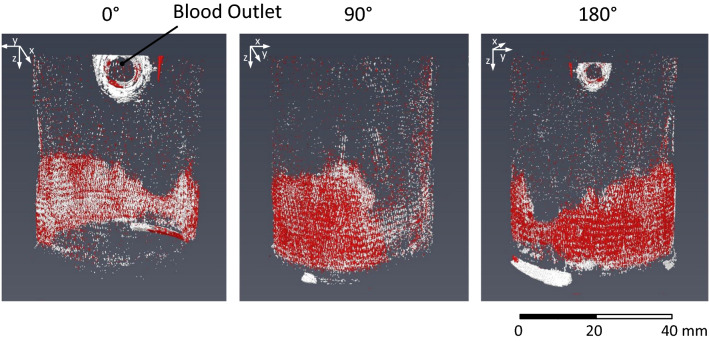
Volume rendering of the 3D subtraction data (CT1-minus-CT8) of Neo2 in 0°, 90°, and 180° rotation angle; higher and lower X-ray intensity in the subtraction data were segmented in red and white color, which relate to red and white thrombus formation, respectively.
